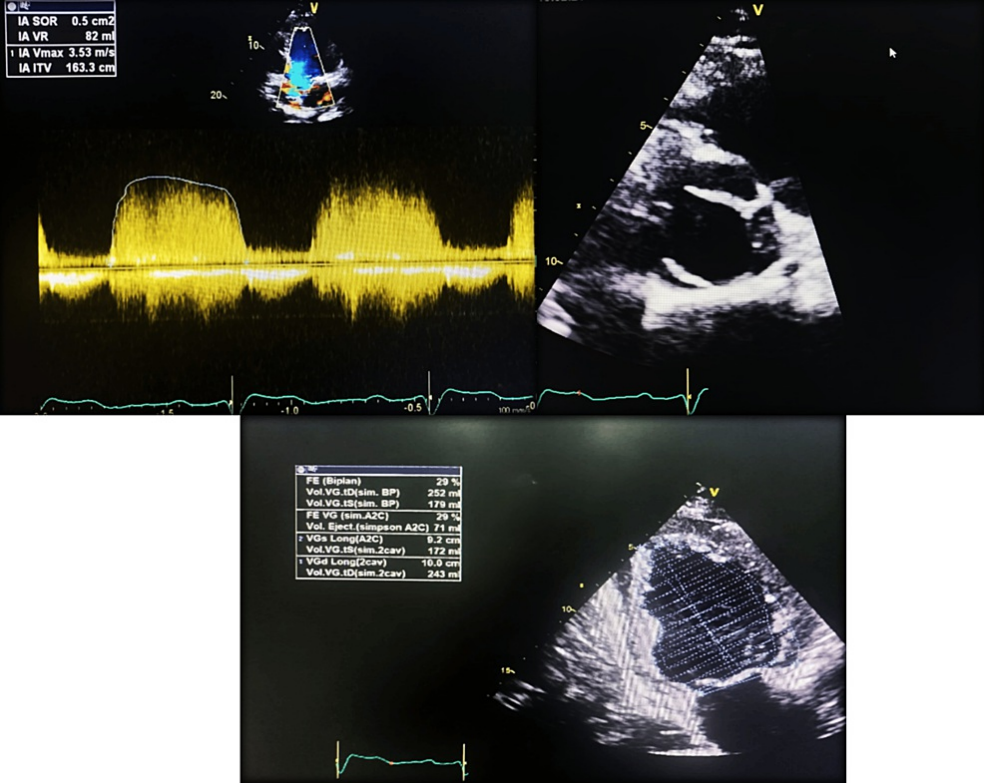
Transthoracic echocardiography showing bicuspid aortic valve with severe aortic regurgitation and left ventricular dysfunction.
